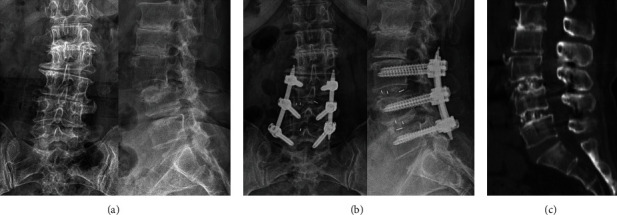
Perioperative plain radiography films. (a) Preoperative anteroposterior and lateral images show degenerative L3–4 spondylolisthesis. (b) Follow-up anteroposterior and lateral films showing reduction of sagittal alignment. (c) Sagittal computed tomography image 14 months postoperatively showing trabecular bridging without endplate disruption or cage subsidence.
